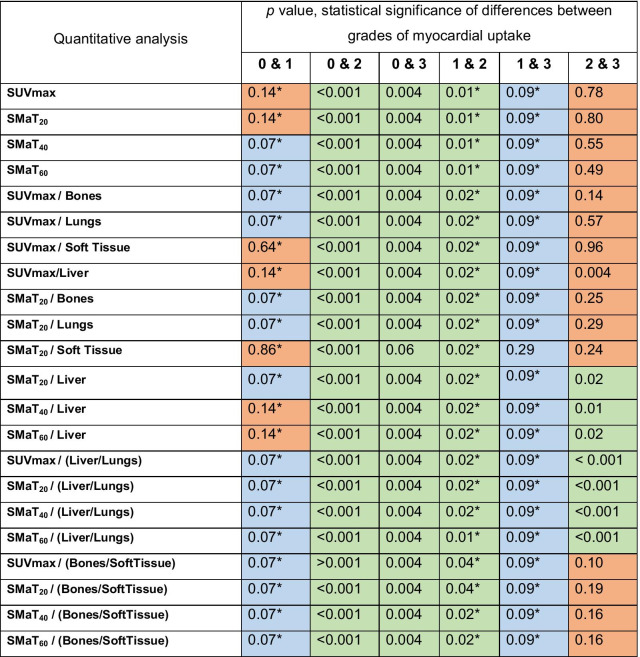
Statistical significance of the difference in SUV values between grades of myocardial uptake
